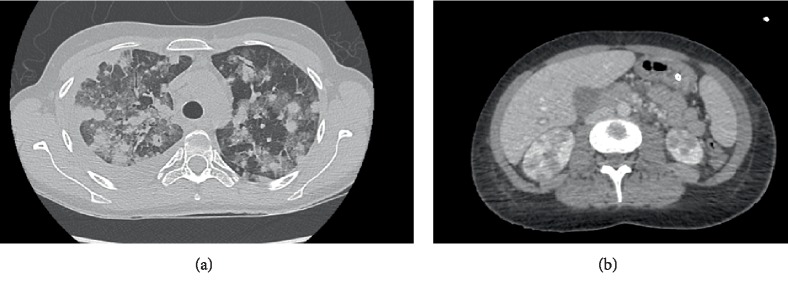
Imaging. (a) Pulmonary computed-tomography (CT) scan showing diffuse bilateral infiltrates. (b) CT with intravenous contrast showing enlargement of both kidneys with bilateral renal nodules.
